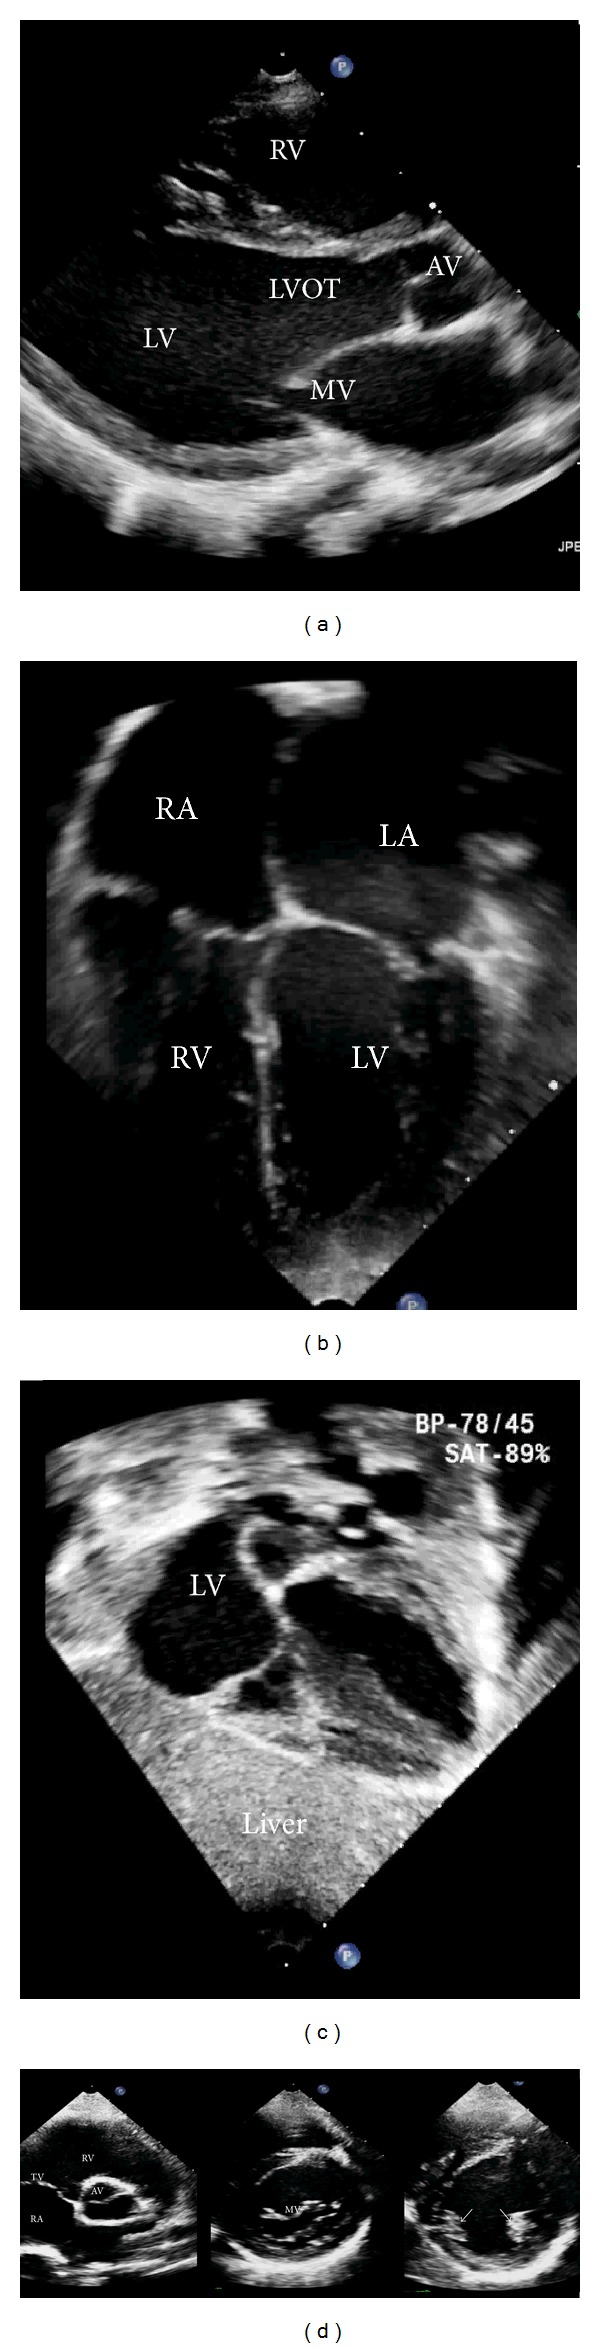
Basic transthoracic echo views: (a) left parasternal long axis, (b) apical, (c) subcostal, and (d) left parasternal short axis; aortic valve “Mercedes sign,” mitral valve “fish mouth sign,” and papillary muscles (two arrows), respectively, from left to right. RV: right ventricle, LV: left ventricle, LVOT: left ventricular outlet, RA: right atrium, LA: left atrium, AV: aortic valve, MV: mitral valve, and TV: tricuspid valve.
